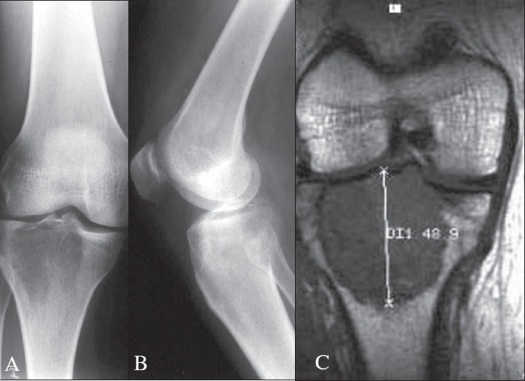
Antero-posterior A) and lateral x-rays. B) with coronal MRI. C) of a GCT of proximal tibia showing a lytic lesion, with sharp, well defined margins and extensive subchondral lysis
